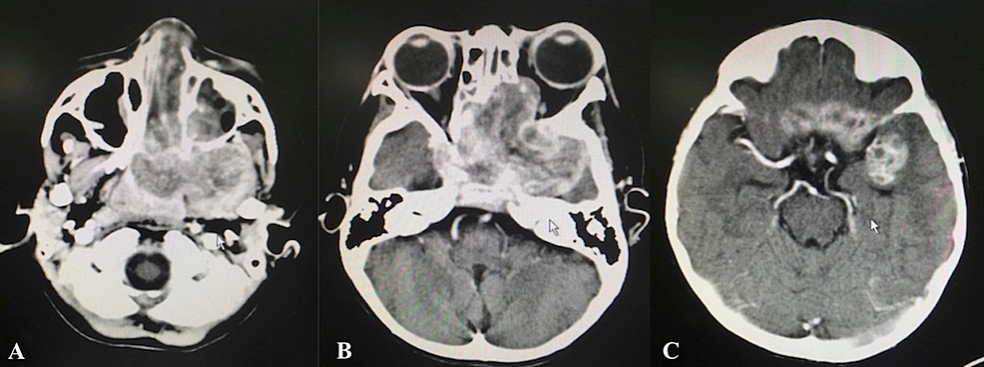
CT scan revealed an extensive sinonasal mass with oropharyngeal (A), intraorbital (B) and intracranial (C) extension.
